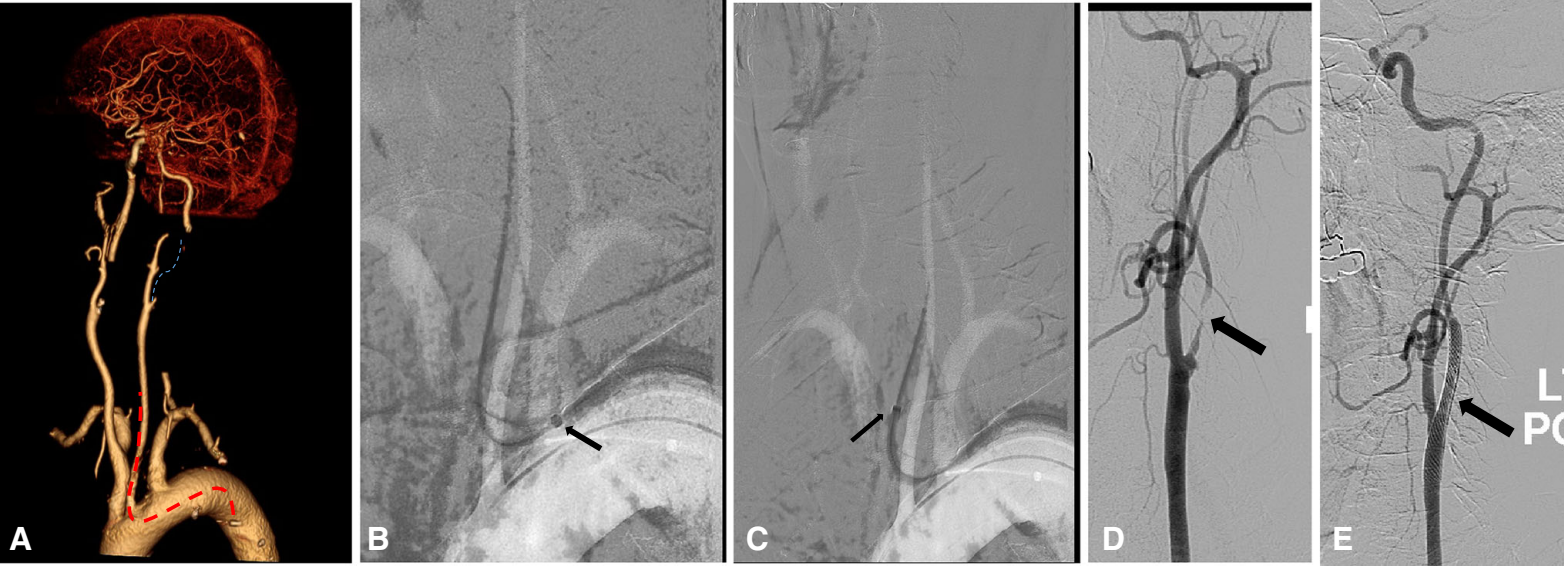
Symptomatic and relatively long left internal carotid artery stenosis. A Volume rendering image of CT angiography showing type III arch, long left internal carotid artery severe stenosis (dotted blue line). The red dotted line shows the expected path of the robotic catheter. B–C Roadmap captures showing the progress path of the robotic catheter (short arrow). D–E Angiography images showing very severe and long internal carotid artery stenosis before and after carotid stent (arrow)
